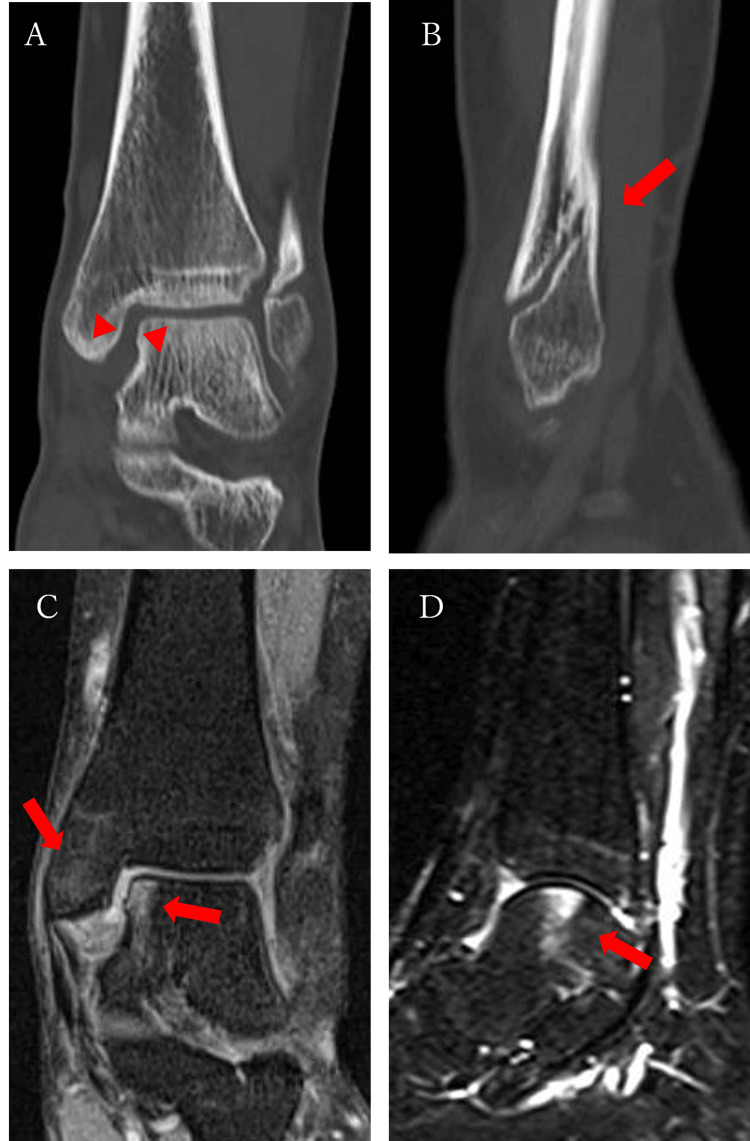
The patient's CT and MRI scans (A) A CT scan (coronal section) shows that the medial joint space has expanded (arrow). (B) A CT scan (sagittal section) shows that the peripheral bone fragments were displaced posterior-superiorly, and there was a gap left in the anterior part of the fracture, but the posterior bone was united (arrow). (C) An MRI proton density-weighted image (coronal section) shows a high signal which was observed on the medial articular surface of the talus and the medial malleolus. (D) An MRI short tau inversion recovery (STIR) image of the sagittal section shows a high signal which was observed on the medial articular surface of the talus and the medial malleolus.
